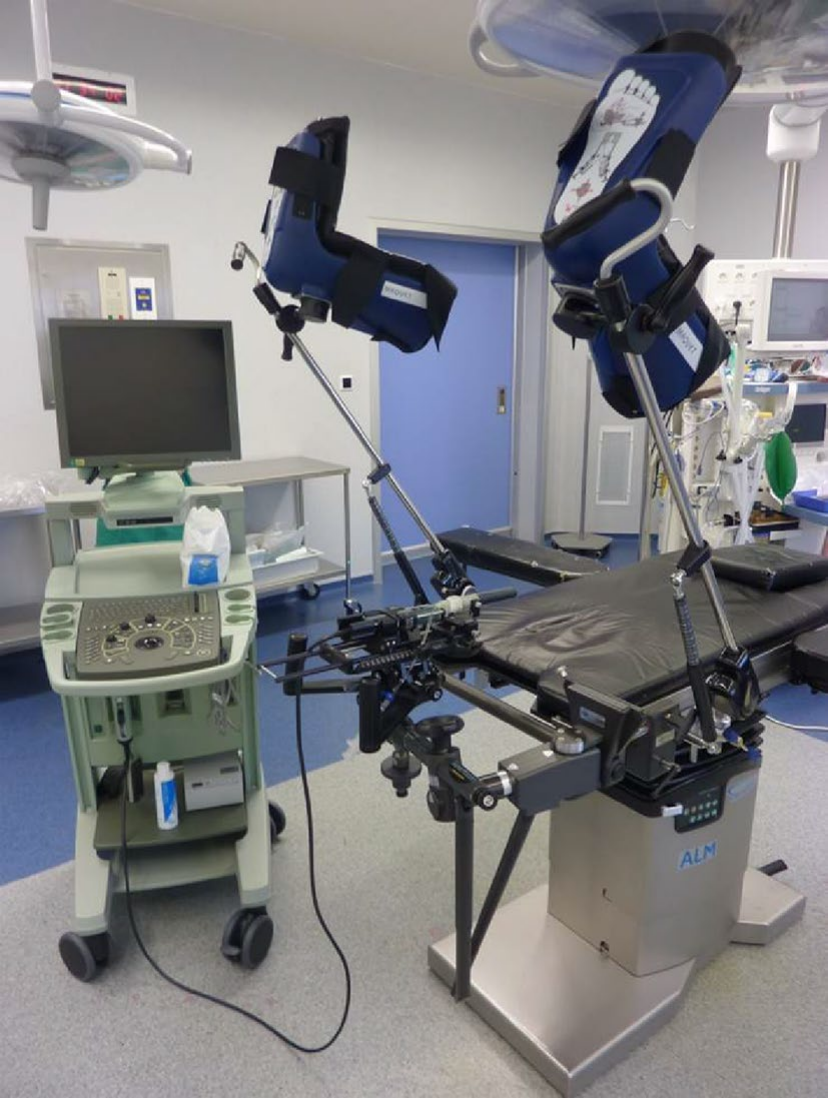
Installation of the operating table, the ultrasound device and the brachystepper
